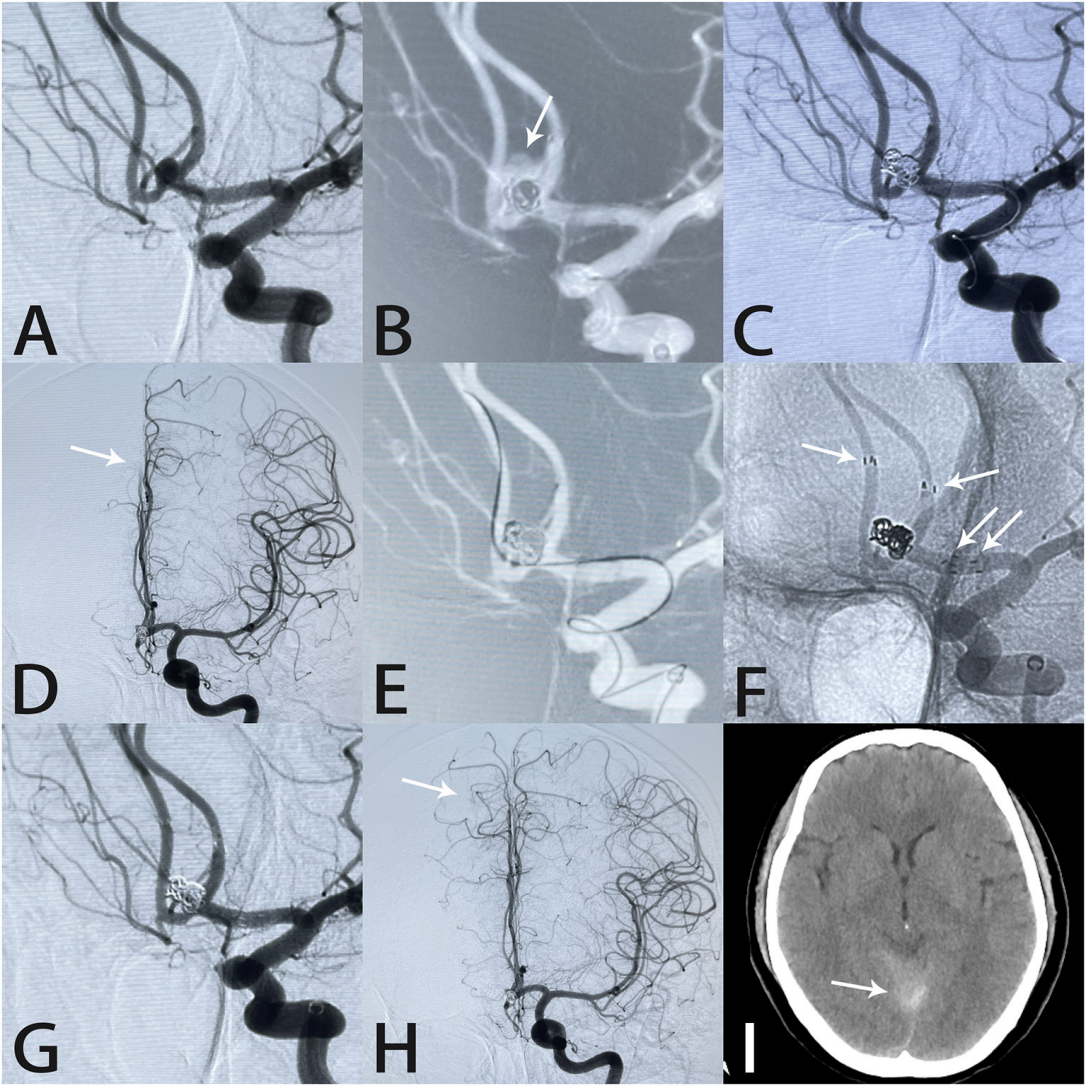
Images from a 56-year-old woman with a tiny anterior communicating artery aneurysm. Angiography demonstrated an anterior communicating artery aneurysm (A). The aneurysm (white arrow) ruptured during coil placement (B). Rapid deployment of additional coils controlled the hemorrhage (C). Intraprocedural angiography showed slow blood flow in the A2 segment of the right anterior cerebral artery (white arrow) (D). An Echelon-10 microcatheter was navigated into the right anterior cerebral artery in preparation for Atlas stent deployment (E). Two Atlas stents formed a “Y-shape” (white arrows) (F). Angiography immediately after the procedure showed complete aneurysm occlusion and unobstructed blood flow in the A2 segment (white arrow) (G,H). Postprocedural computed tomography showed subarachnoid hemorrhage in the tentorium cerebelli (I).
